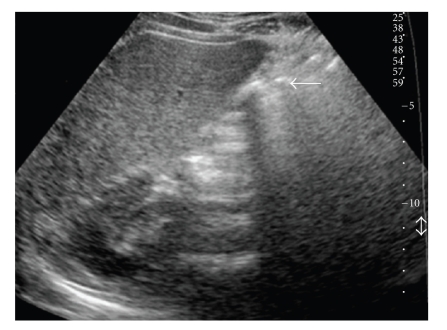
Ultrasonography scan demonstrates an hyperechoic area with an acoustic shadow in the gallbladder fosse (arrow). These findings usually are suspected for sclero-atrophic or contracted lithiasic gallbladder.
